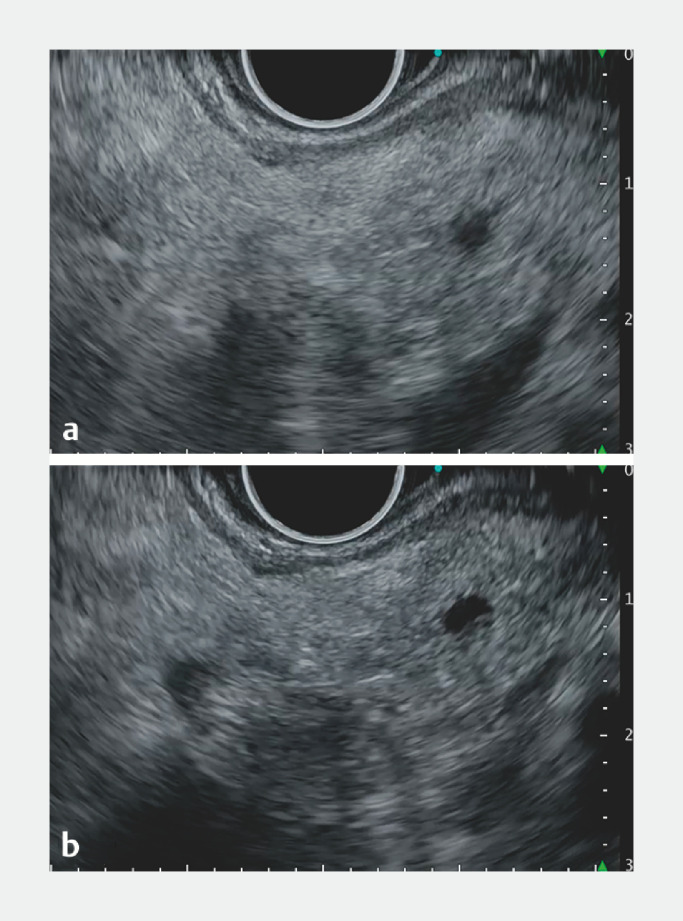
Endoscopic ultrasound (EUS) images of the main pancreatic duct (1.8 mm in diameter) closer to the anastomosis during EUS-guided pancreatography drainage.
